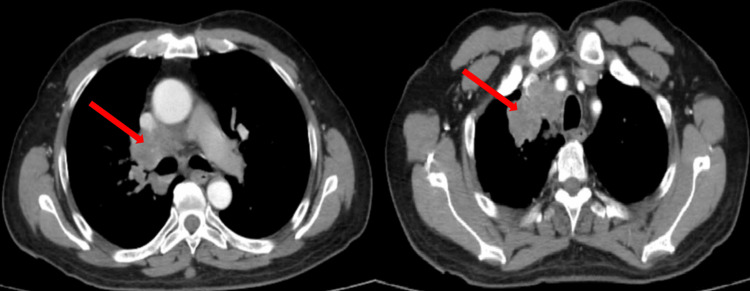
CT chest sections of the patient. Pulmonary tumoral process of the apicoventral segment of the right upper lobe of 45×42×42 mm in large axes (arrows), with homolateral medial ganglionic magma of 75 mm in his posterior-anterior diameter with bilateral pulmonary micronodules of suspicious appearance.
